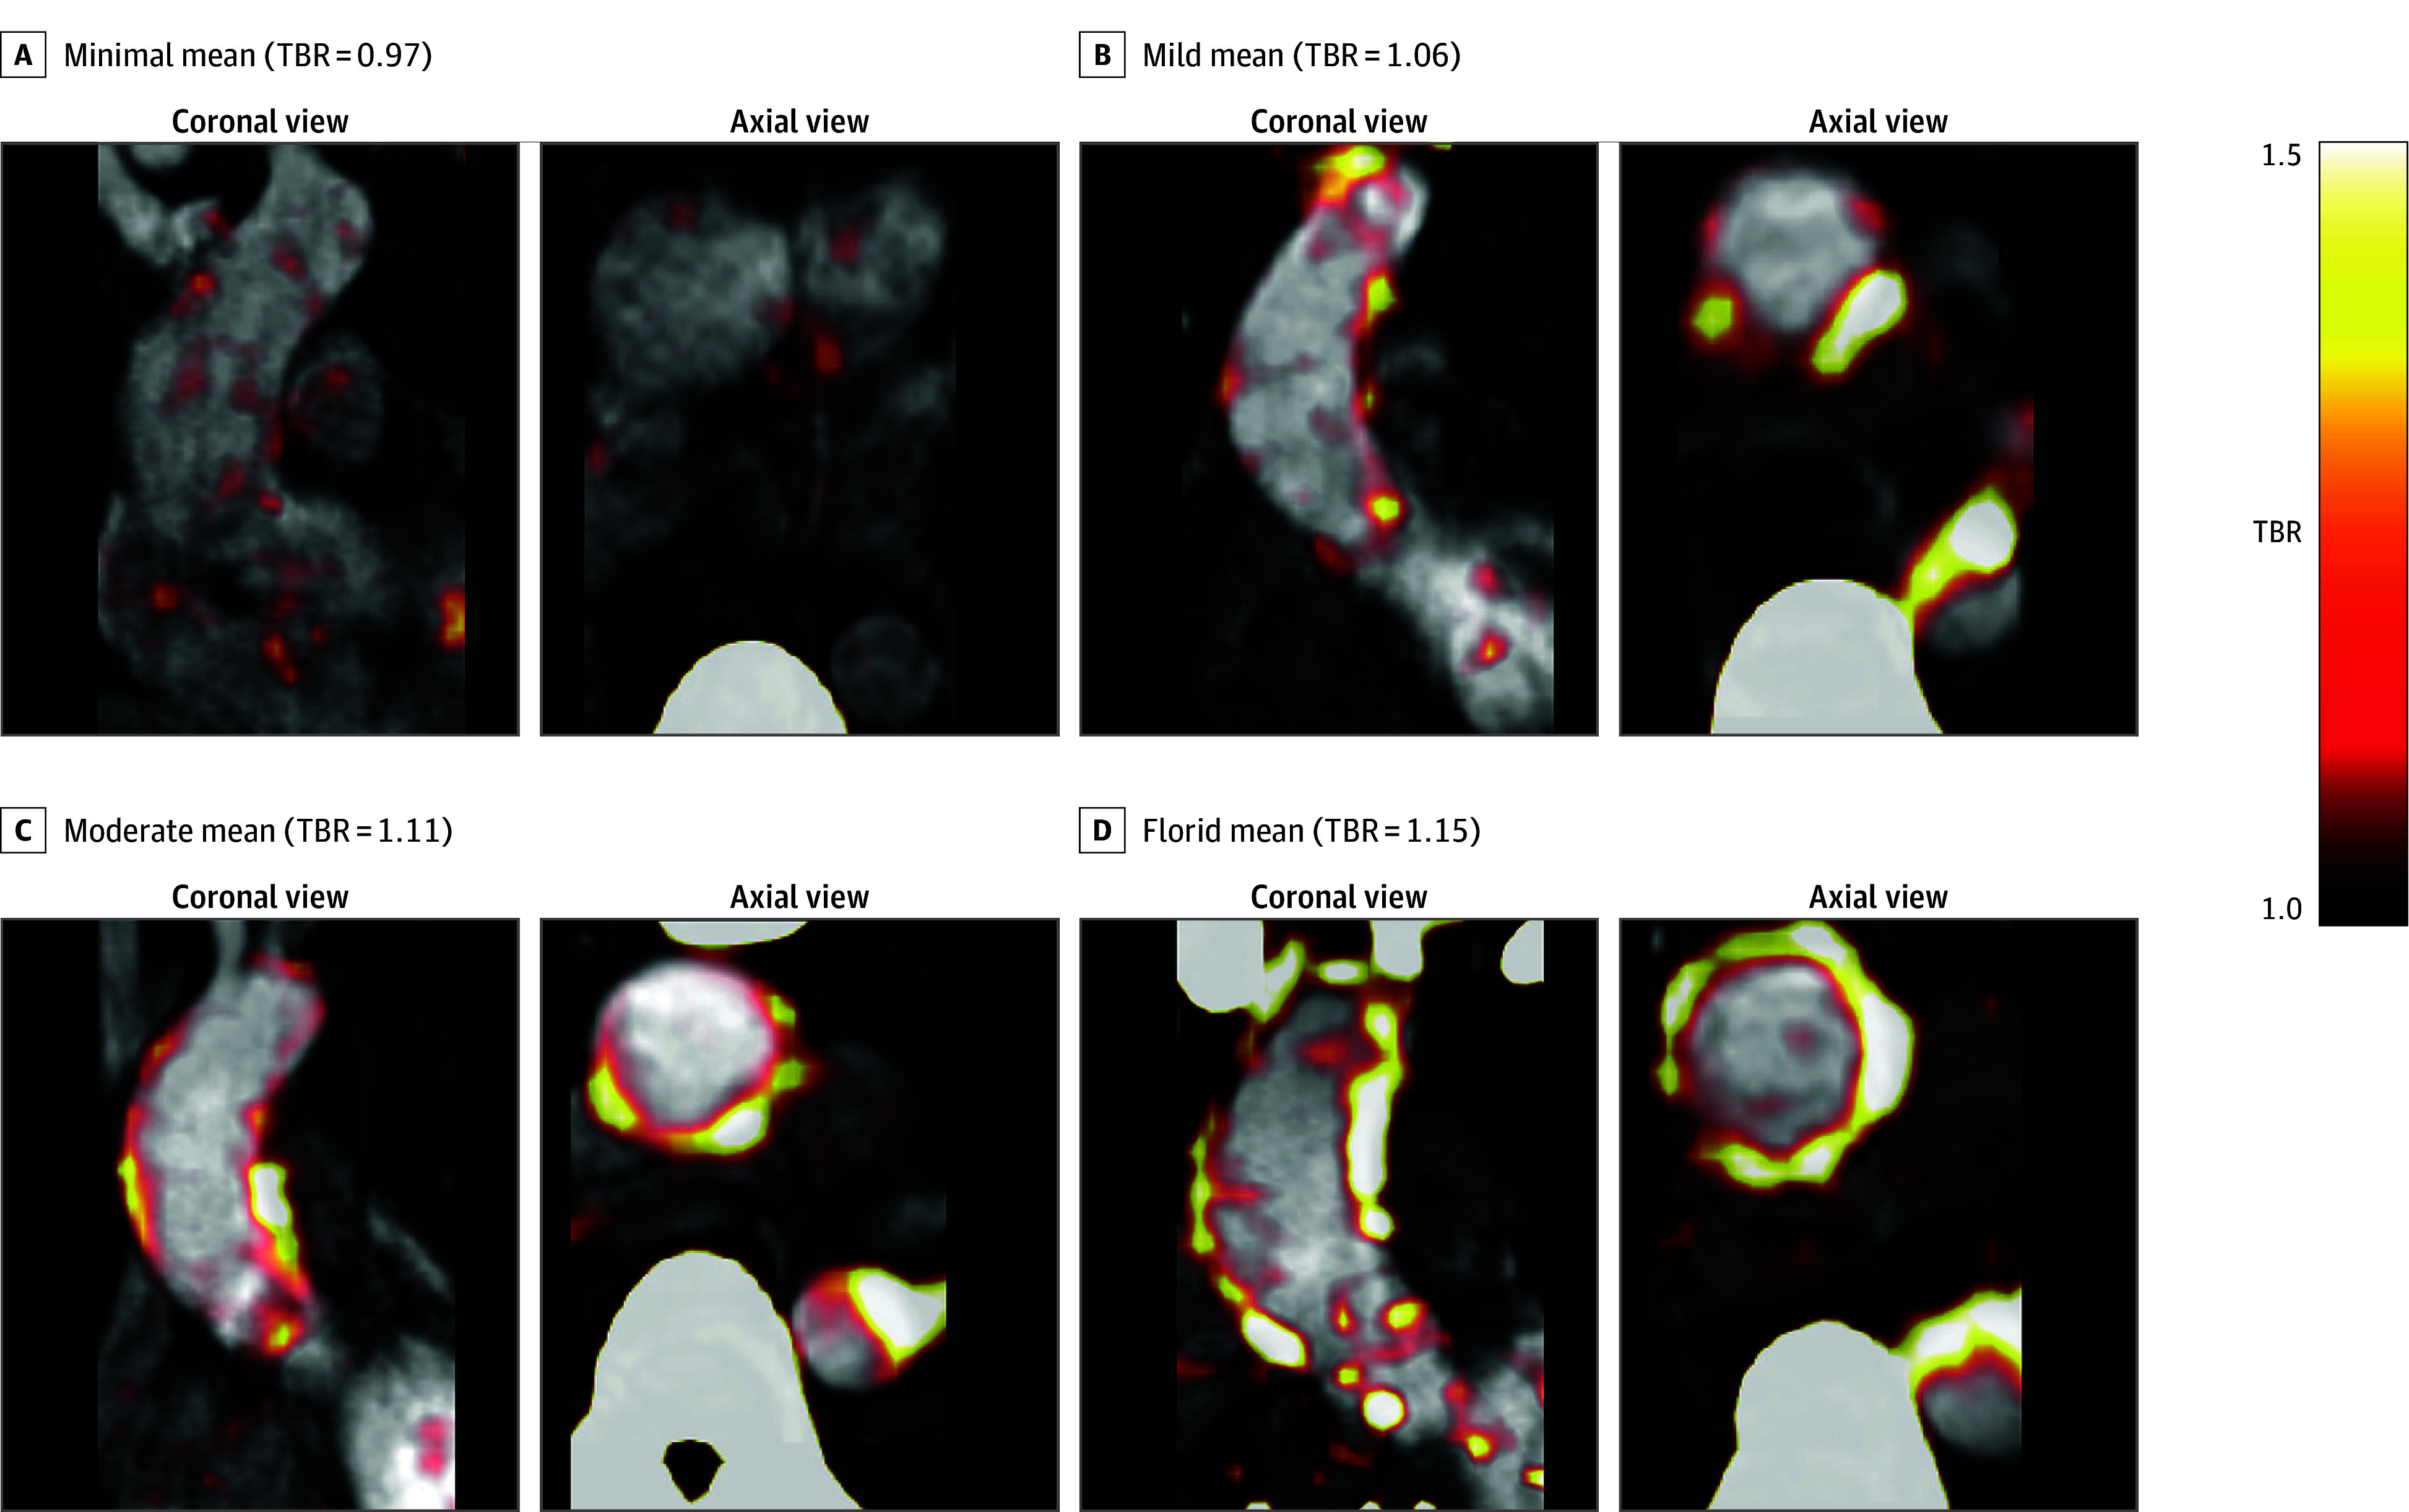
Fluorine F 18–Labeled ([18F])–Sodium Fluoride Uptake in the Ascending Aorta Coronal and axial [18F]–sodium fluoride positron emission tomography with computed tomography attenuation correction fused with magnetic resonance angiogram. Examples are shown for minimal (A), mild (B), moderate (C), and florid (D) ascending aortic [18F]–sodium fluoride uptake. Each positron emission image is scaled to a tissue background ratio of 1 to 1.5. TBR indicates tissue to background ratio.
